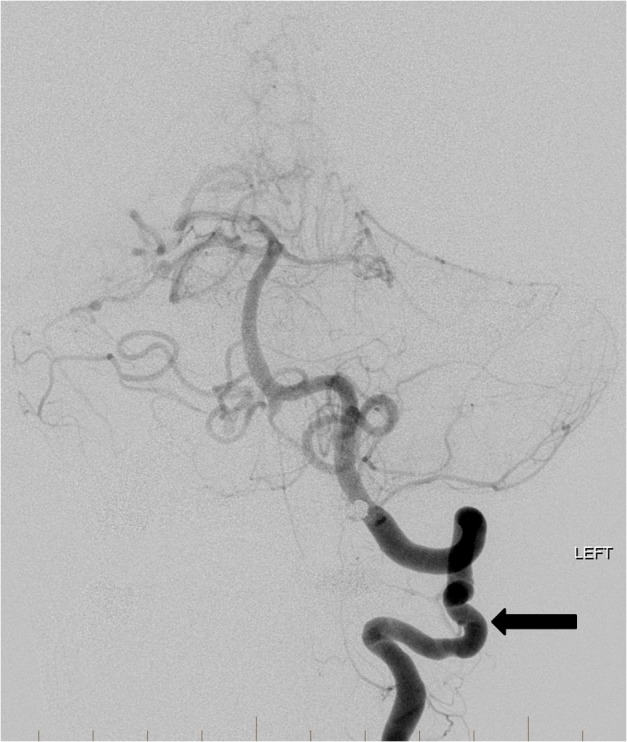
Frontal projection left vertebral artery angiogram was used as a roadmap for puncture of the vertebral artery (arrow) while simultaneously visualizing under ultrasound guidance. "Left" indicates the patient's left side.
